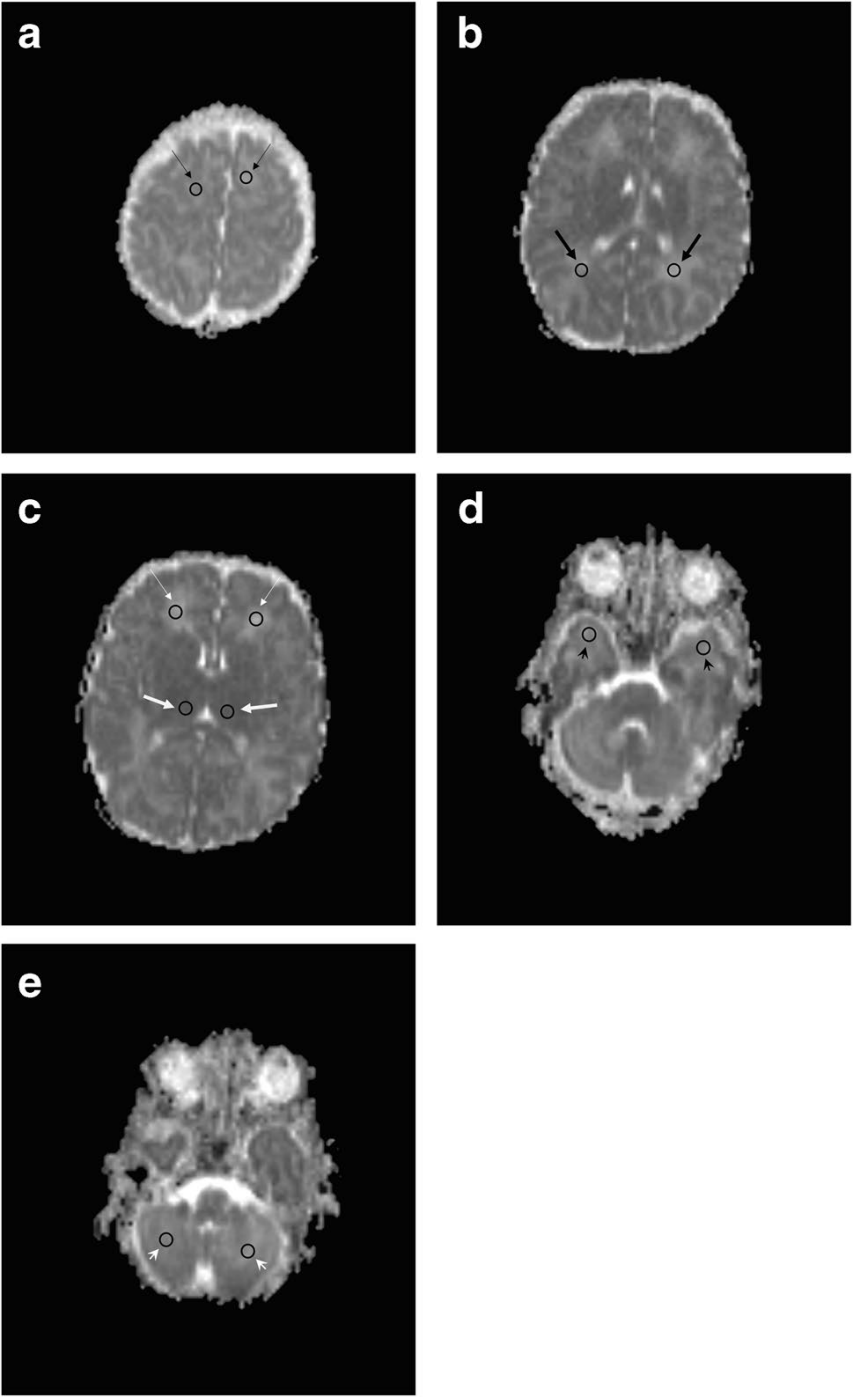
Axial apparent diffusion coefficient magnetic resonance images in a 2-week-old boy with congenital cytomegalovirus infection show placement of regions of interest (*arrows*) in the subcortical frontoparietal white matter (a), posterior periventricular white matter (b), deep anterior frontal white matter (*thin arrows* in c), thalami (*thick arrows* in c), anterior temporal white matter (d), and cerebellar hemispheres (e)
